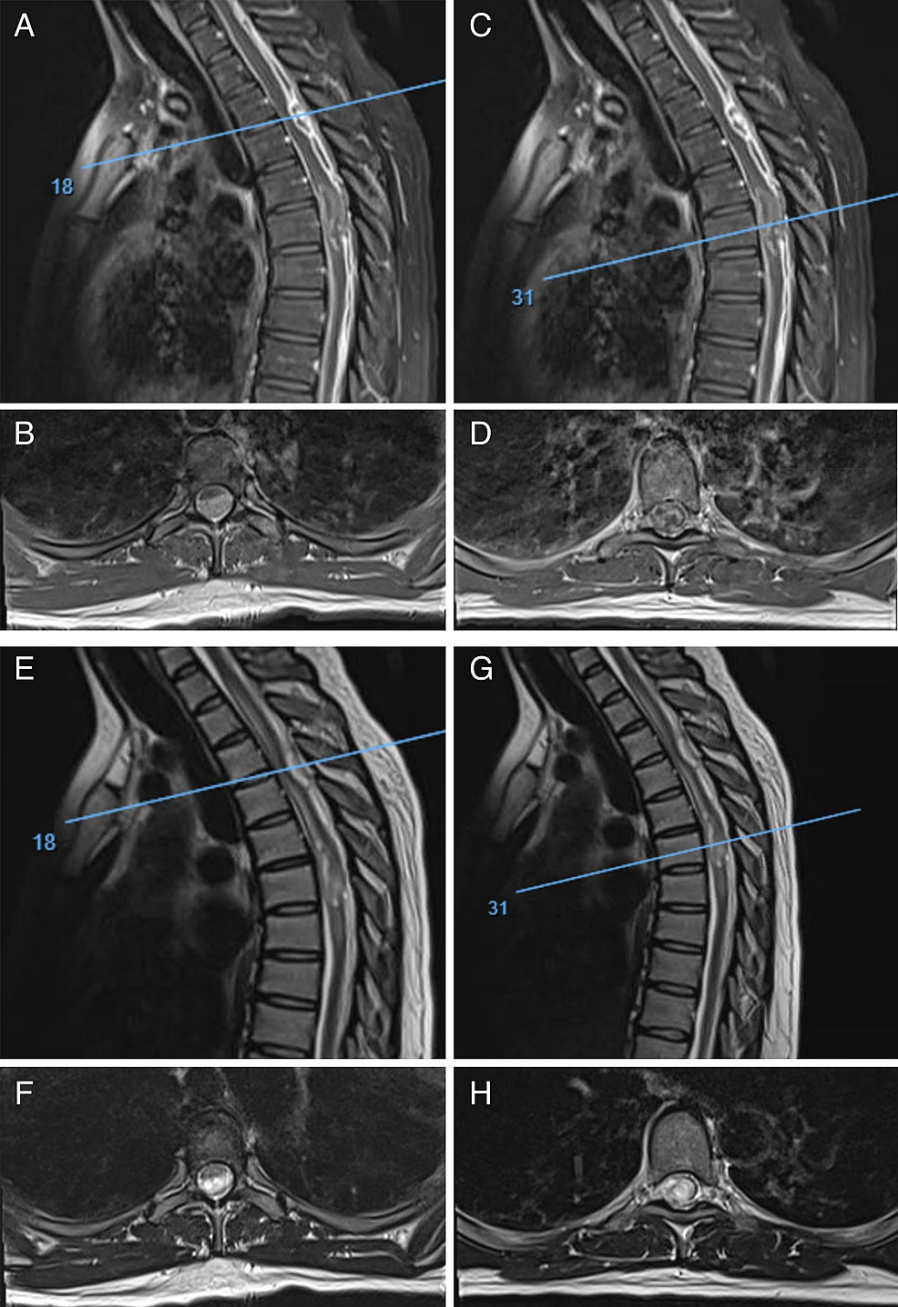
(A) and (B) T1-weighted sagittal MRI with IV contrast of the thoracic spine demonstrating the diffuse, heterogeneous leptomeningeal enhancement with loculated dorsal mass impinging the thecal sac (A) and intramedullary, exophytic mass (C). (B) Axial image of the slice indicated in (A) by the blue line. (D) Axial image of the slice indicated in (C) by the blue line. (E) and (G) T2-weighted sagittal MRI without contrast. (F) Axial image of the slice indicated in (E) by the blue line. (H) Axial image of the slice indicated in (G) by the blue line MRI, Magnetic resonance imaging; IV, intravenous.
